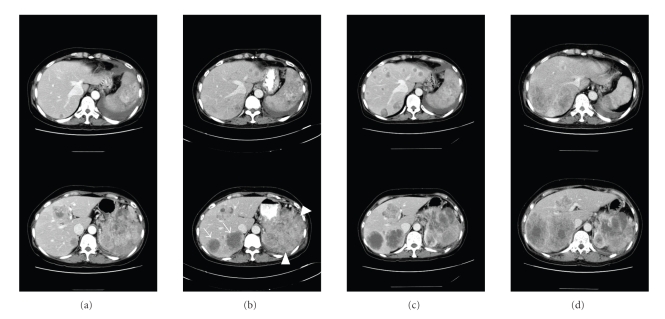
Serial CT scans show variable response in retroperitoneal mass (arrowhead), multiple nodules of left hepatic lobe and two nodules (arrows) of right posterior lobe. (a) At initial presentation, (b) after one cycle of weekly paclitaxel, (c) after two cycles of doxorubicin, (d) after 3 months of sunitinib.
